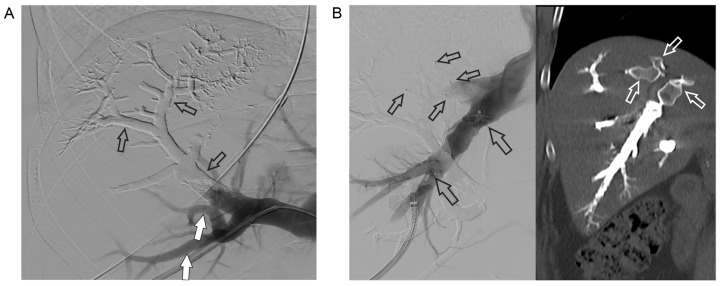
A. PVE: a part of the right portal vein and the branches of segments 7 and 8 (open arrows) are already filled with embolization material (Glubran 2/Lipiodol), in contrast, the percutaneous accessed branches of segments 5 and 6 are not embolized but opacified with contrast media at the time of DSA (open arrows); B. occlusion of the right hepatic vein (left): venogram of the percutaneously punctured right hepatic vein. An Amplatzer vascular plug 2 with 16 mm diameter (large open arrows) has already been detached. Small open arrows point at 2 other vascular plugs detached in two (non-opacified) tributaries of the right hepatic vein. Post-intervention CT in the coronal plane (right) shows the vascular plugs (open arrows) and the right hepatic vein completely filled with embolization material. DSA: digital subtraction angiography
